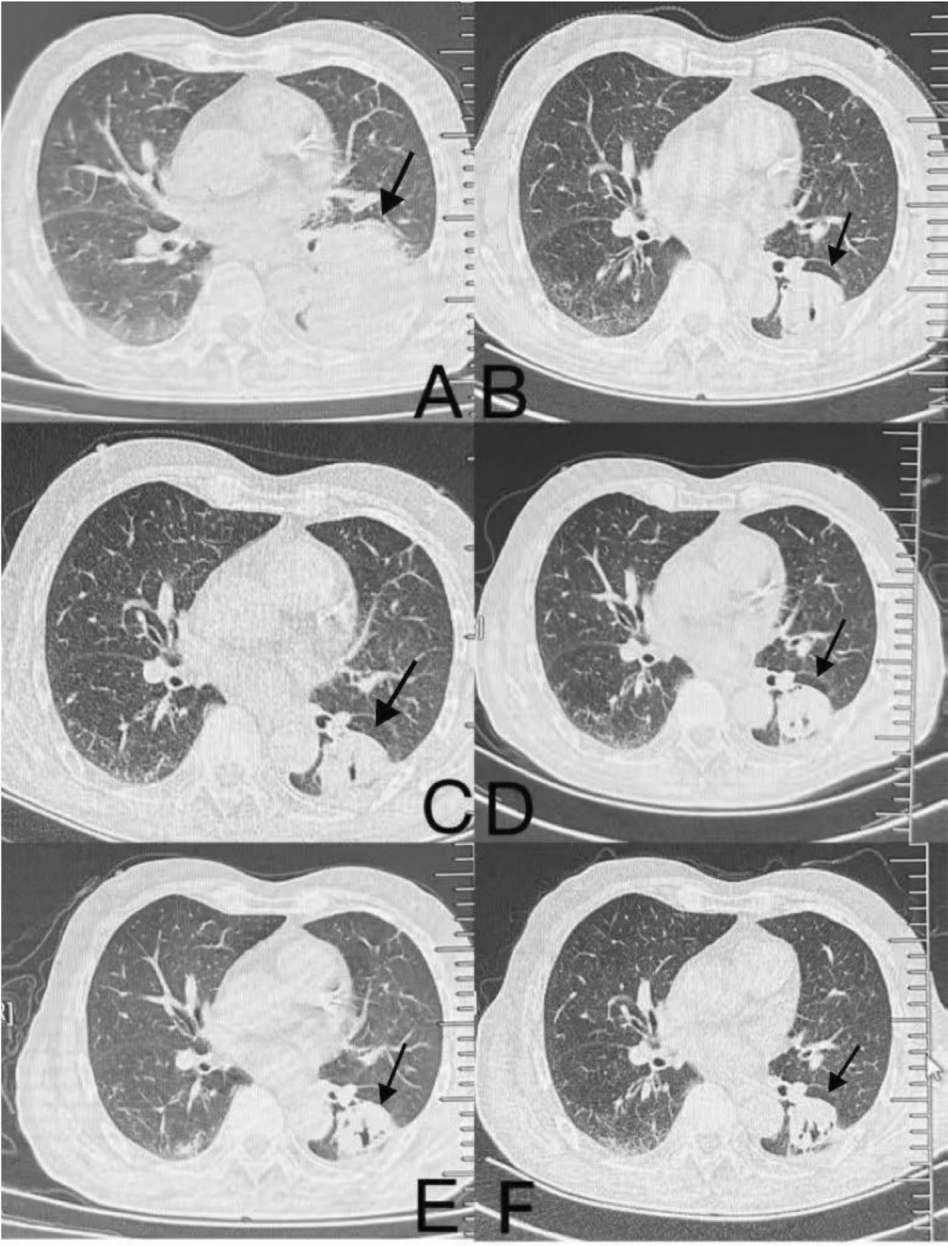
Changes under CT scan. The lung CT scan from 2023/6 to 2024/4 revealed the patient's left pulmonary mass was reduced by more than 30%, with uneven density and cavitation. 2023/6/22 Chest CT: A mass of soft tissue density shadow was observed in the lower lobe of the left lung, with uneven density and a diameter of approximately 64 mm. The adjacent bronchi were compressed and narrowed, and the pleura was invaded (A); 2023/12/22 Chest CT: A mass shadow of approximately 33 × 39 mm could be seen in the lower lobe of the left lung, with a cavity within it (B); 2024/1/22 Chest CT: A mass shadow could be seen in the lower lobe of the left lung, approximately 35 mm × 40 mm, slightly larger than before, with a cavity within it (C); 2024/2/26 Chest CT: A mass shadow of approximately 30 mm × 27 mm could be seen in the lower lobe of the left lung, with a cavity within it (D); 2024/3/21 Chest CT: A mass image of approximately 29 mm × 25 mm could be seen in the lower lobe of the left lung, with a cavity within it, and the cavity is larger than before (E); 2024/4/21 Chest CT: A mass image of approximately 26 mm × 23 mm could be seen in the lower lobe of the left lung, with a cavity within it, and the cavity is larger than before (F).
